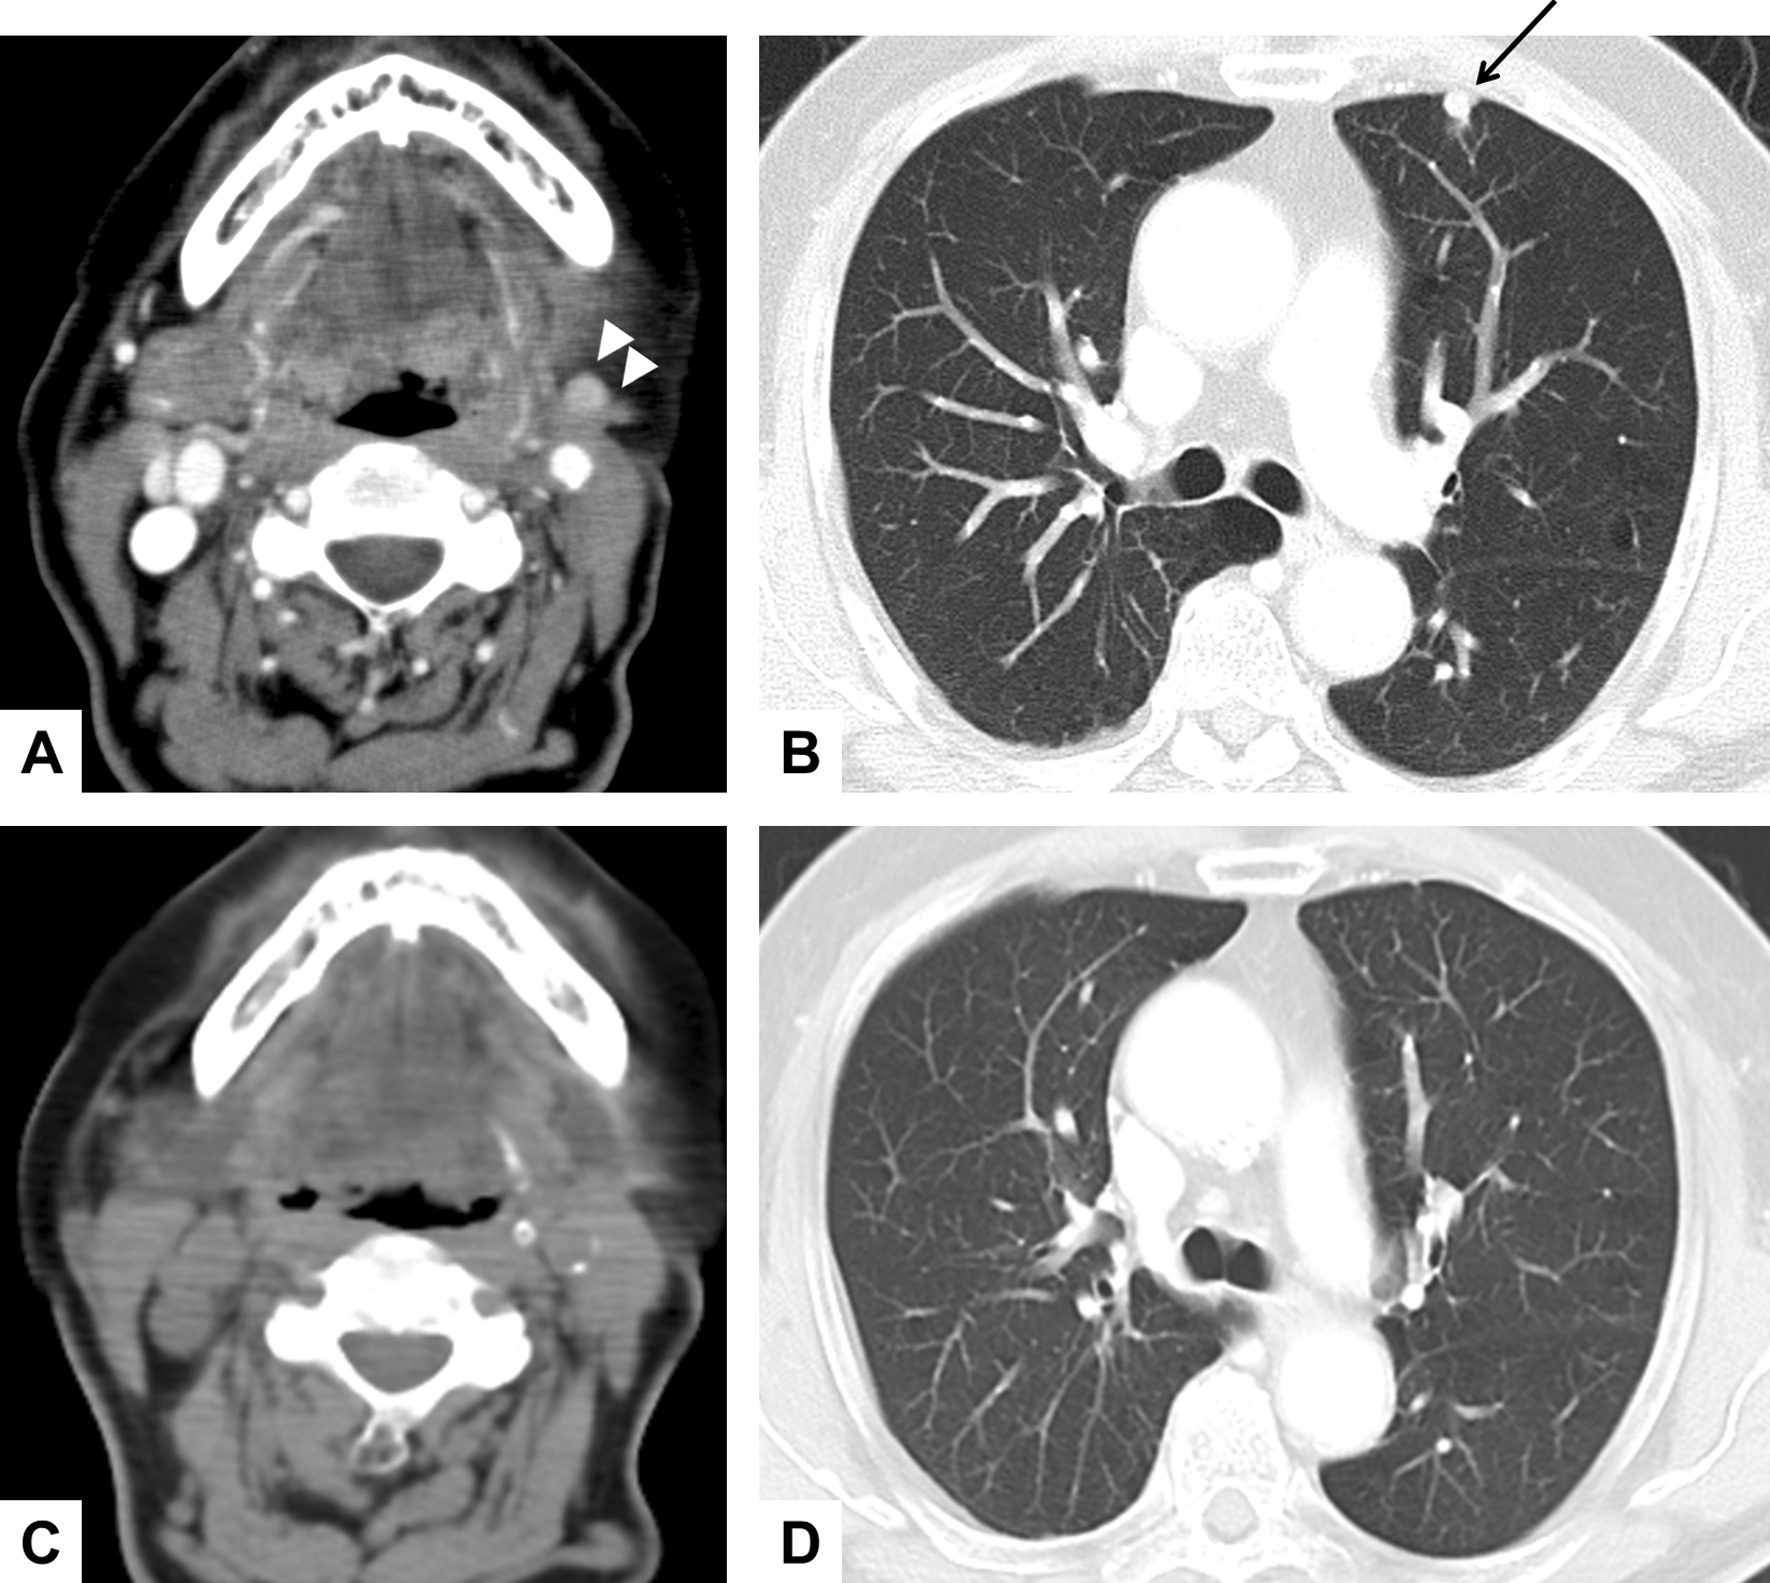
A durable complete response after entrectinib therapy in a SC patient with regional recurrence and pulmonary metastasis (Case 2). CT imaging of the patient before (A and B) and at 18 months after (C and D) entrectinib therapy are shown. Arrow heads indicate regional recurrence and the black arrow indicates pulmonary metastasis
